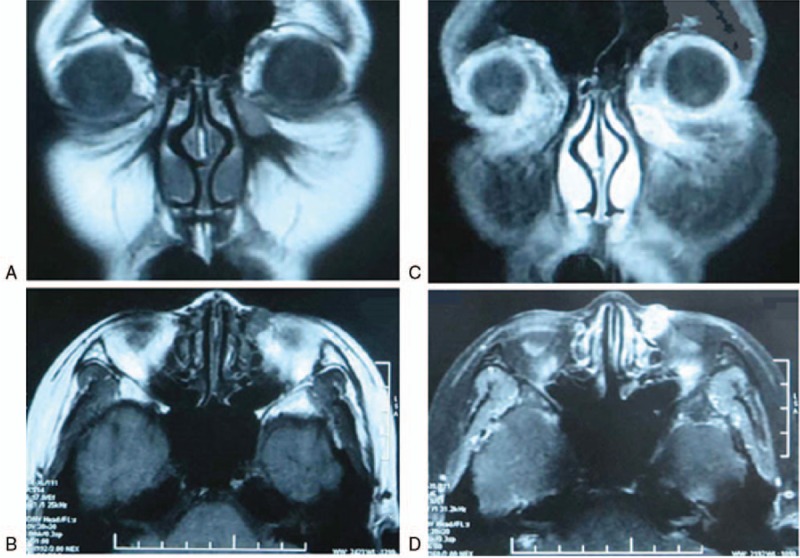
Magnetic resonance imaging (MRI) scan, demonstrating (A) Axial and (B) coronal sections of MRI scan and (C), (D) enhancement showing a tumor (10 mm×12 mm) with nonuniform density in the area of left dacryocystitis.
